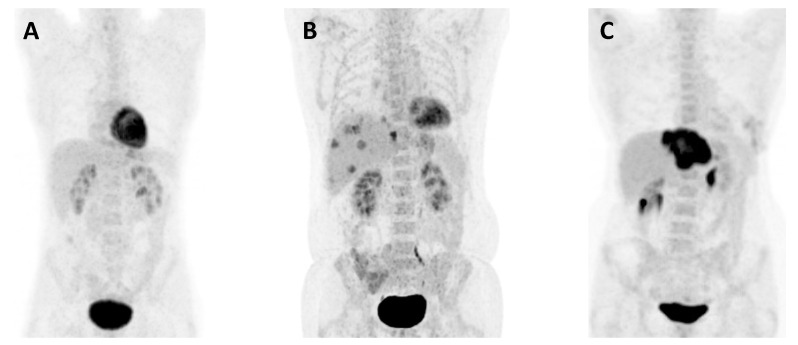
Image examples. (A) This patient had a low MTV (0 cm^3^) and 40 liver metastases on the CT, and the largest metastasis was 38 mm with a TBS of 40.2. The patient is still alive more than 12 years after LT. (B) This patient had a low MTV (46.35 cm^3^), 25 liver metastases on CT, the largest metastasis was 35 mm, and a TBS of 25.2. The patient is still alive more than 5 years after LT. (C) This patient had a high MTV (146.50 cm^3^), 7 liver metastases on the CT, the largest metastasis was 105 mm, and a TBS of 12.6. The patient died only 2 years after LT.
